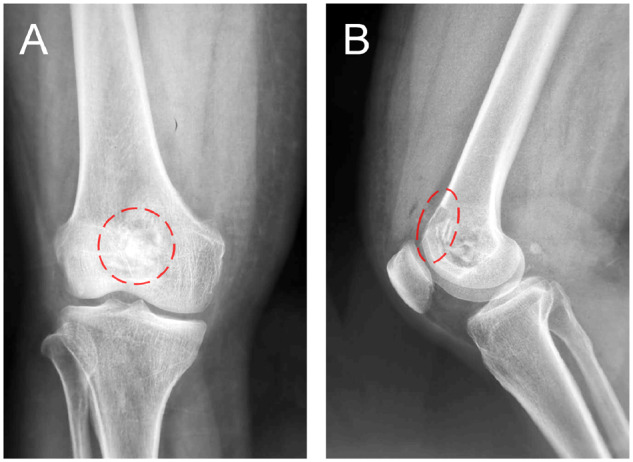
Immediate frontal (A) and lateral (B) views of plain radiography after the operation (the surgical site is noted by dashed circle in each picture).
